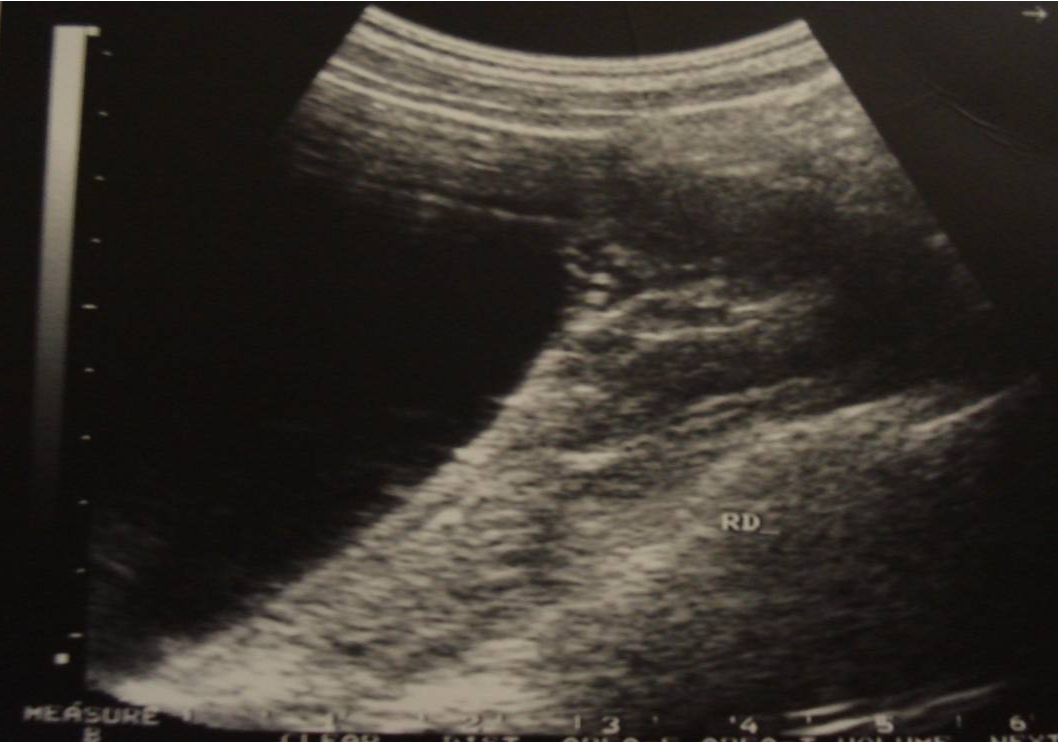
Ultrasonography showing a giant right renal cyst.
